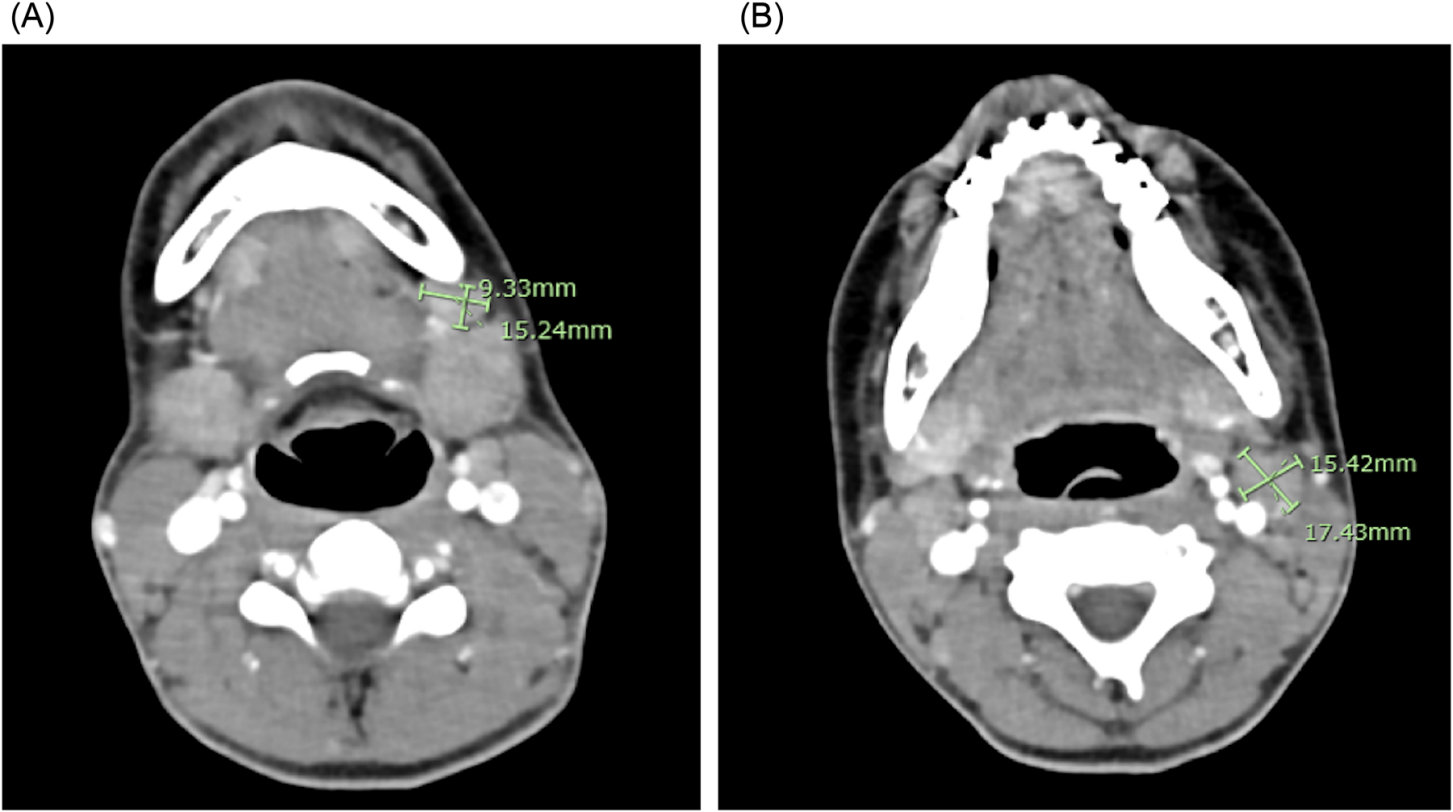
Contrast CT neck/chest. (A) Bilateral submandibular masses with largest left‐sided mass measuring 15 × 9 mm. (B) Mildly enlarged bilateral jugulodigastric lymph nodes with left node measuring 17 × 15 mm.
